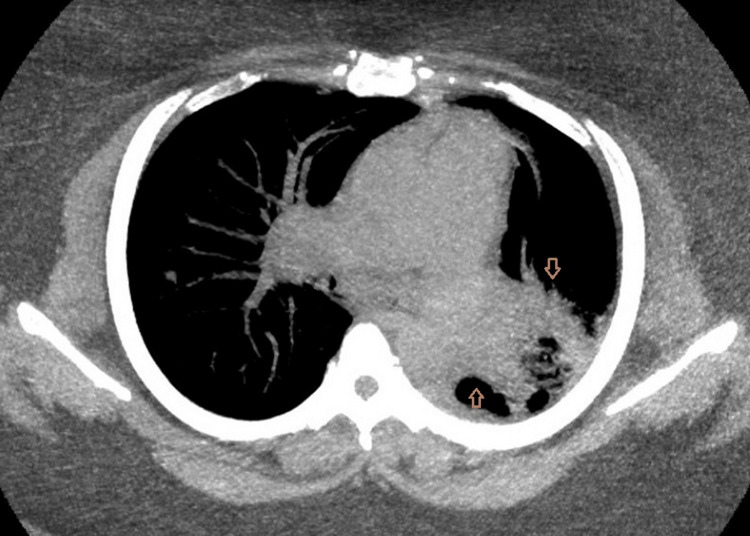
Axial soft tissue window CT image demonstrating heterogeneous left-sided consolidation with expansion and internal gas pockets, along with mild rightward mediastinal shift, concerning for necrotic consolidation (arrows).
